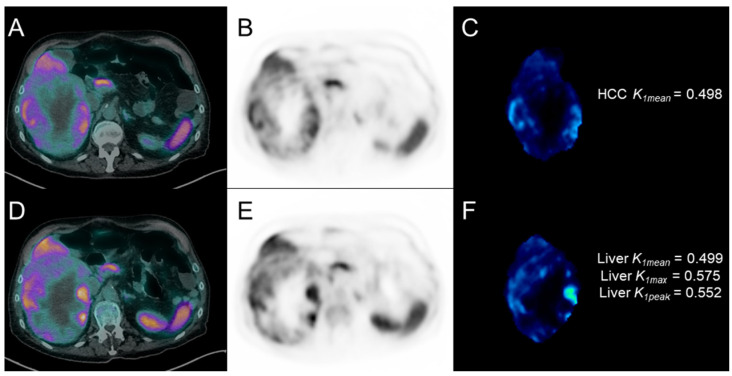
^13^N-ammonia PET images of patient #16, with a wide HCC lesion of the right liver lobe, at baseline ((A): transaxial PET/CT; (B): transaxial PET; (C): *K_1_* parametric map of HCC lesion) and at post-treatment ((D): transaxial PET/CT; (E): transaxial PET; (F): *K_1_* parametric map of non-neoplastic liver parenchyma).
